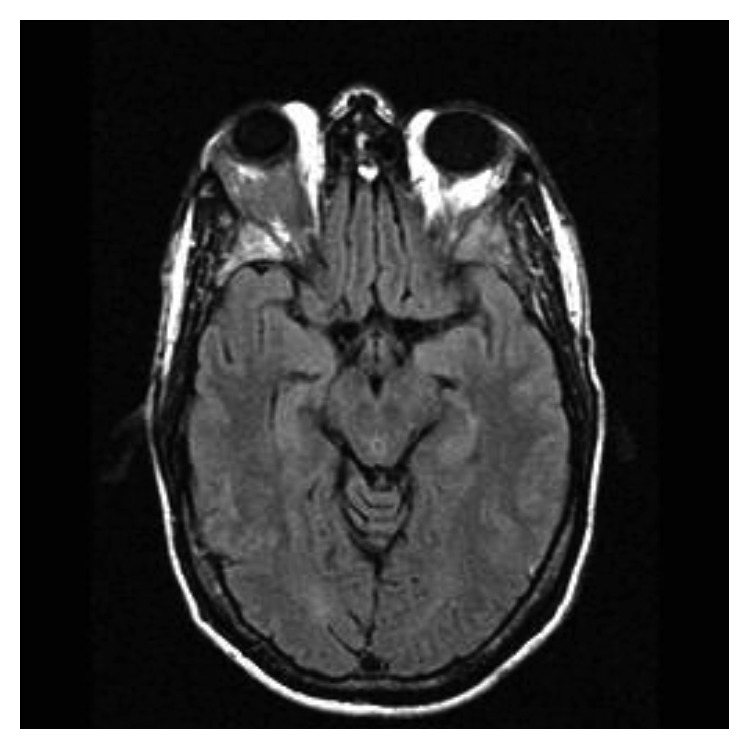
MRI after KRd treatment: gradual reduction in size of the soft-tissue plasmacytomas in the absence of local RT.
